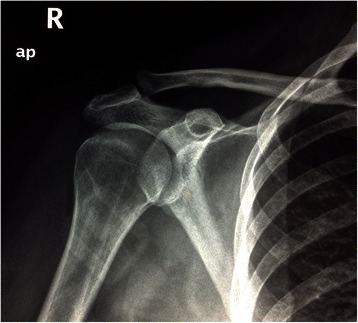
Anteroposterior X-ray of the patient’s right shoulder demonstrating mild proximal elevation of the head and moderate arthritis in her acromioclavicular joint
